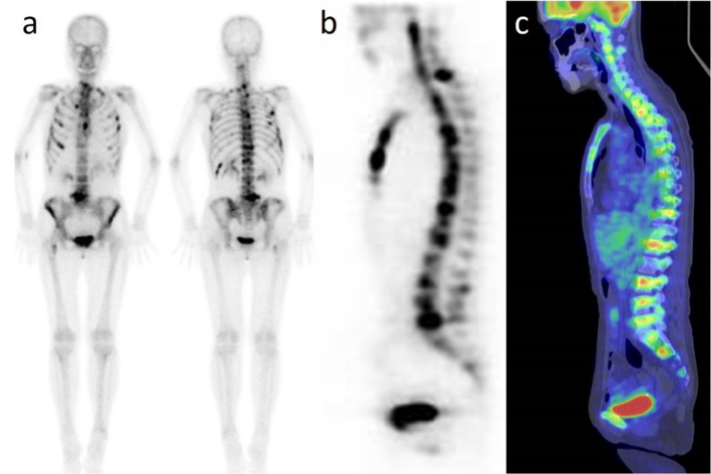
a, b Bone scintigraphy and c fluorodeoxyglucose-positron emission tomography of the patients. Multiple bone metastases to the thoracic to lumbar vertebrae, costae, sternum, iliac bone, scapulae, and femora are shown, suggestive of disseminated carcinomatosis of the bone marrow
